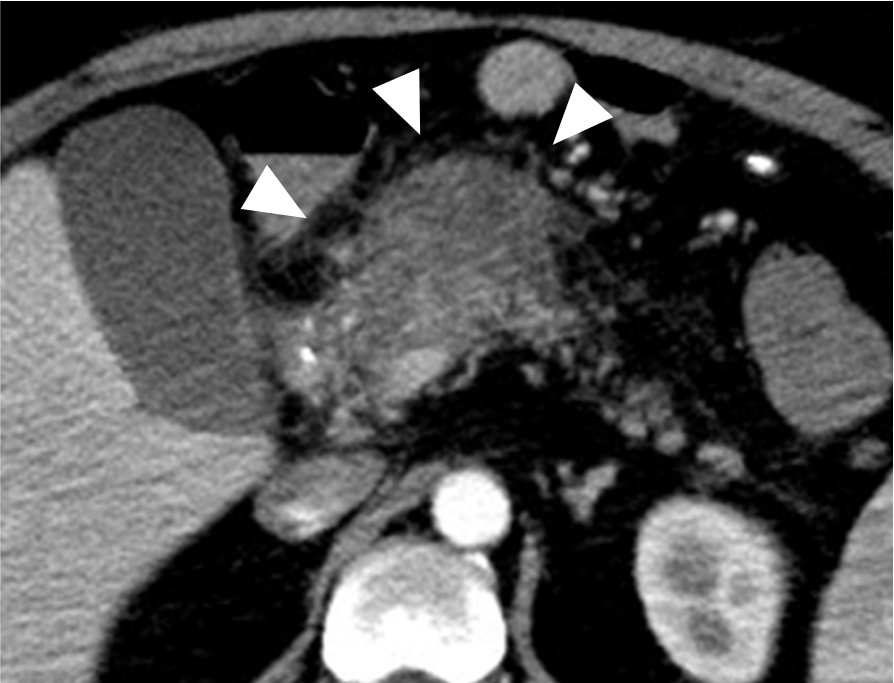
Abdominal plain computed tomography (CT) findings. Plain CT on POD5 showing fluid collection in the anterior cavity of the pancreatic head (arrow)
